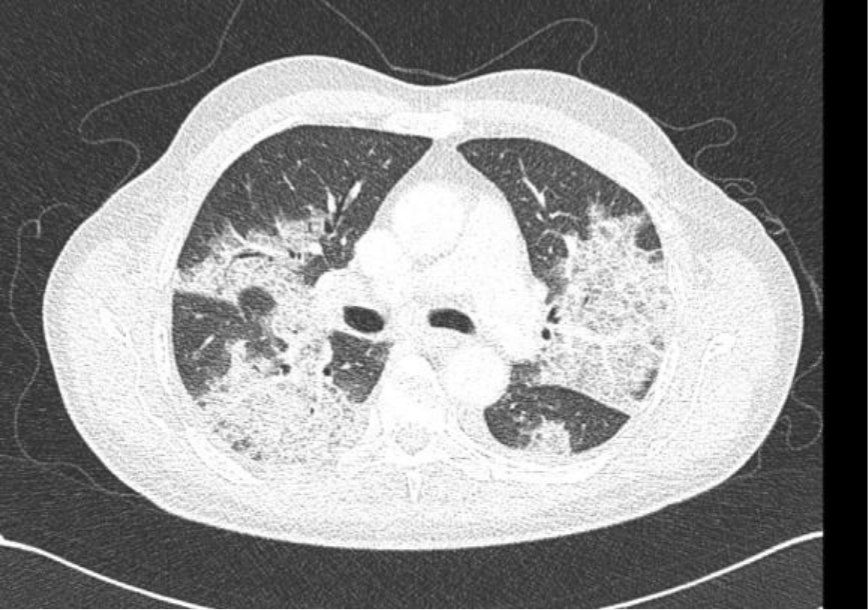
Case #1: CT image showing bilateral upper lobe consolidation.
